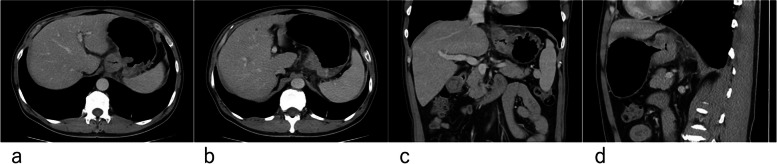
a-d, a patient with poorly differentiated HAS at the junction of esophagus and stomach. Enhanced CT showed full-thickness involvement of the gastric wall with the involvement of the surrounding fat space, increased density of the surrounding fat space, and visible cord shadow. The postoperative pathology showed vascular tumor thrombus was positive. Multiple liver metastasis occurred 18 months after radical surgery
